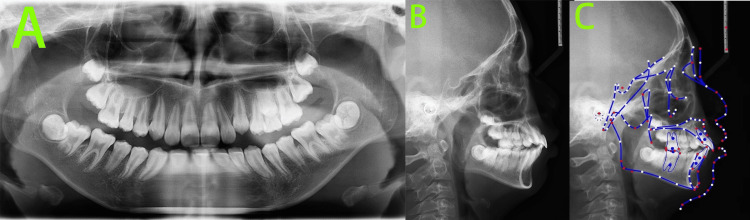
Pre-phase II radiographs A: Panoramic radiograph revealed root resorption of tooth 65; B: Lateral cephalogram; C: Cephalometric tracing indicated significant retraction of the upper anterior teeth and an improvement in the skeletal Class II relationship. Cephalometric tracing in Figure 5C created using the UCeph software (version 3.1, UCeph Software, Chengdu, China).
